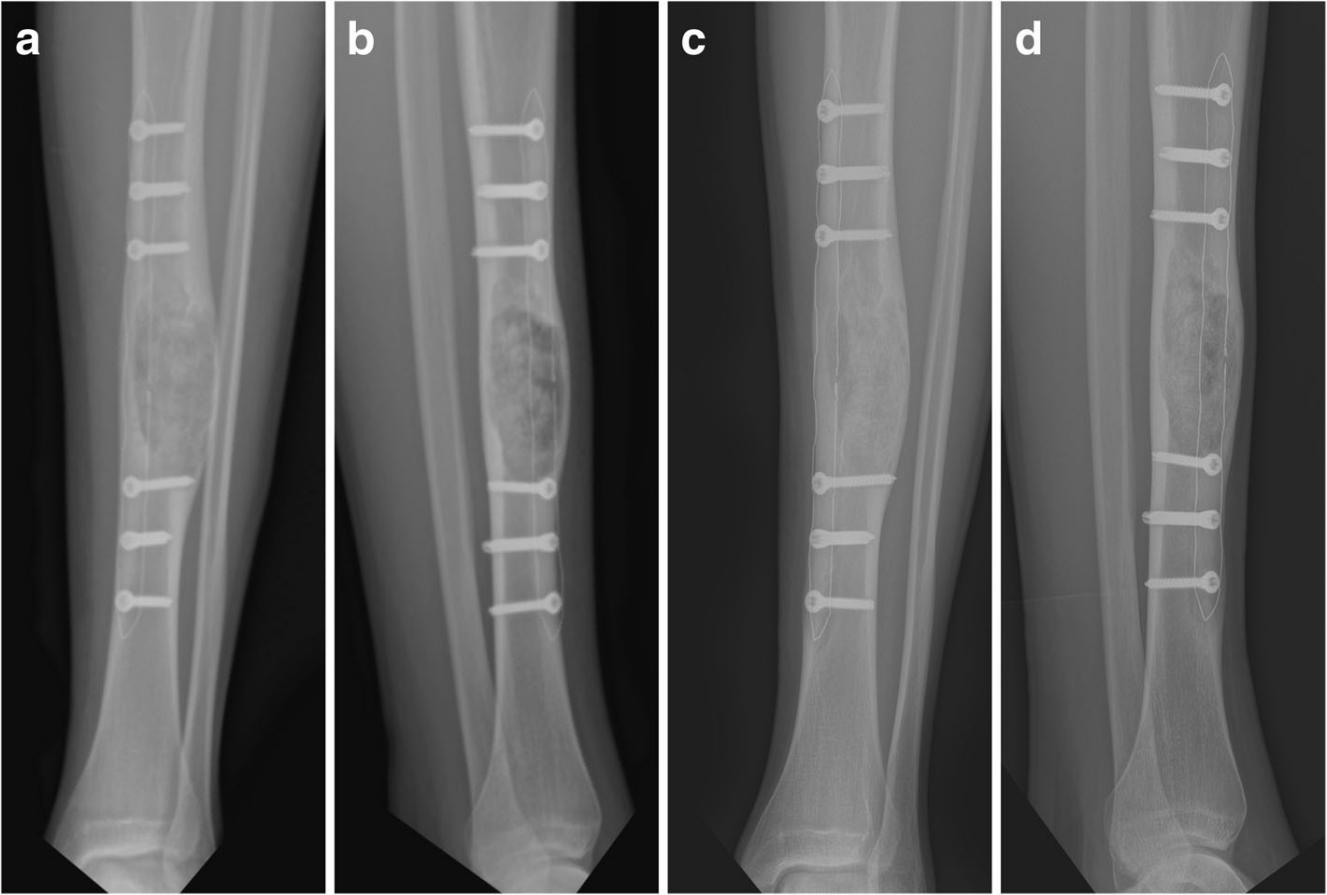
Postoperative radiographs with excellent assessability of bone healing after 6 weeks (a, b) and 6 months (c, d)
